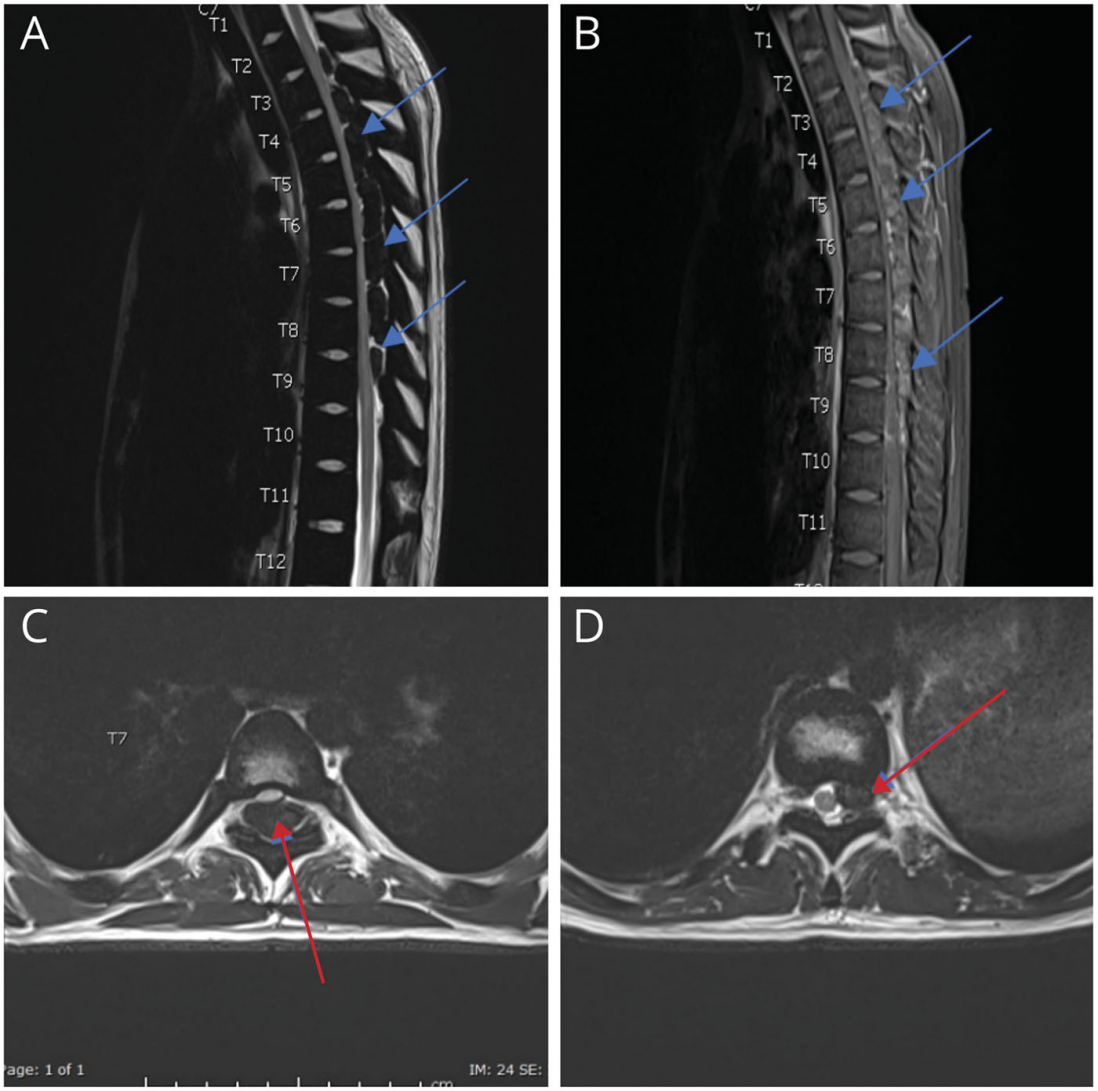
MRI spine: intraspinal epidural extramedullary masses T2 (lowered border) to T9 (arrows in A and B). Anterior displacement and compression of the cord in the thoracic spine is secondary to the compression by the EMH (arrow in C at T7 level) reaching upto the left neural foramen (arrow in D at T10 level). *Note*. Image taken from Clinical Case reports from a previously published article [18]. The licence of the article (CC-BY) permits unrestricted reuse of the published work.
